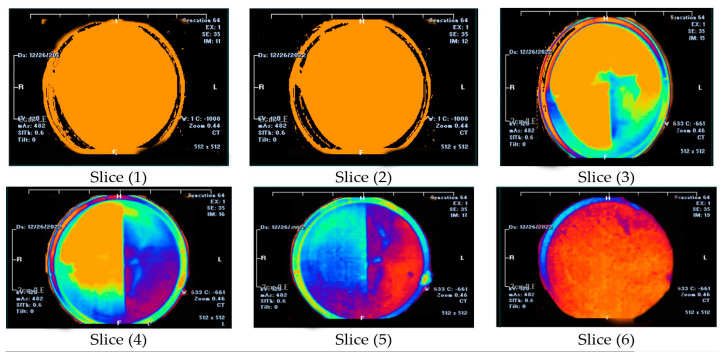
The CT scan images for the mud cake samples containing 2 wt.% MNPs. The CT scan images were taken in the direction of the filtrate invasion. Slice 1 represents the top surface of the mud cake, whereas slice 6 represents the bottom surface.
